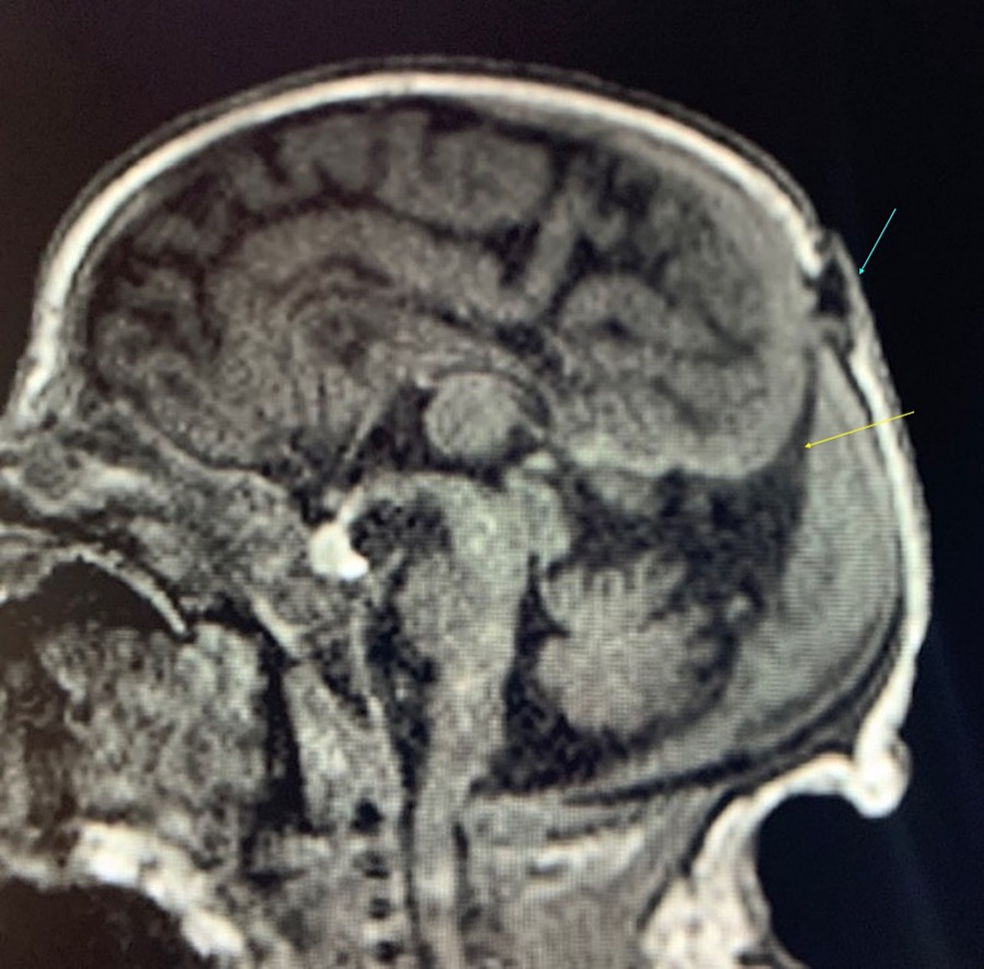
Sagittal FLAIR pre-contrast MRI. The image is showing superior beaking of the tentorium (yellow arrow) as it approaches the scalp lesion (blue arrow). FLAIR: fluid-attenuated inversion recovery
